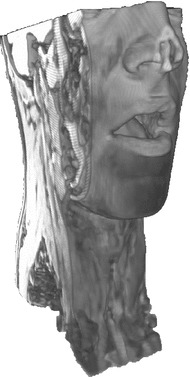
Example of a three-dimensional stack based on MRI recordings, where the subject produces the vowel /a/ in singing mode
